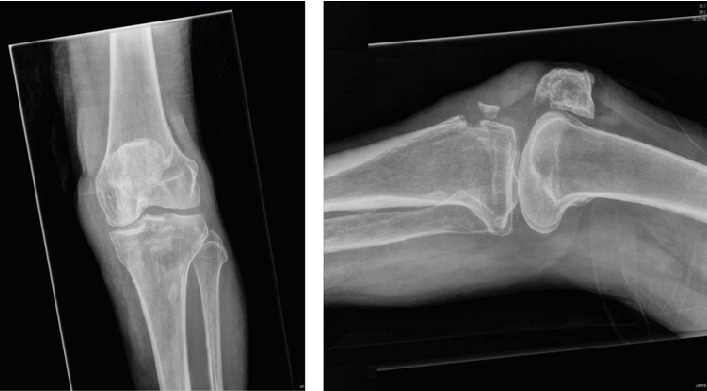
Left knee AP and lateral radiographs showing avulsion fracture of the tibial tuberosity.
